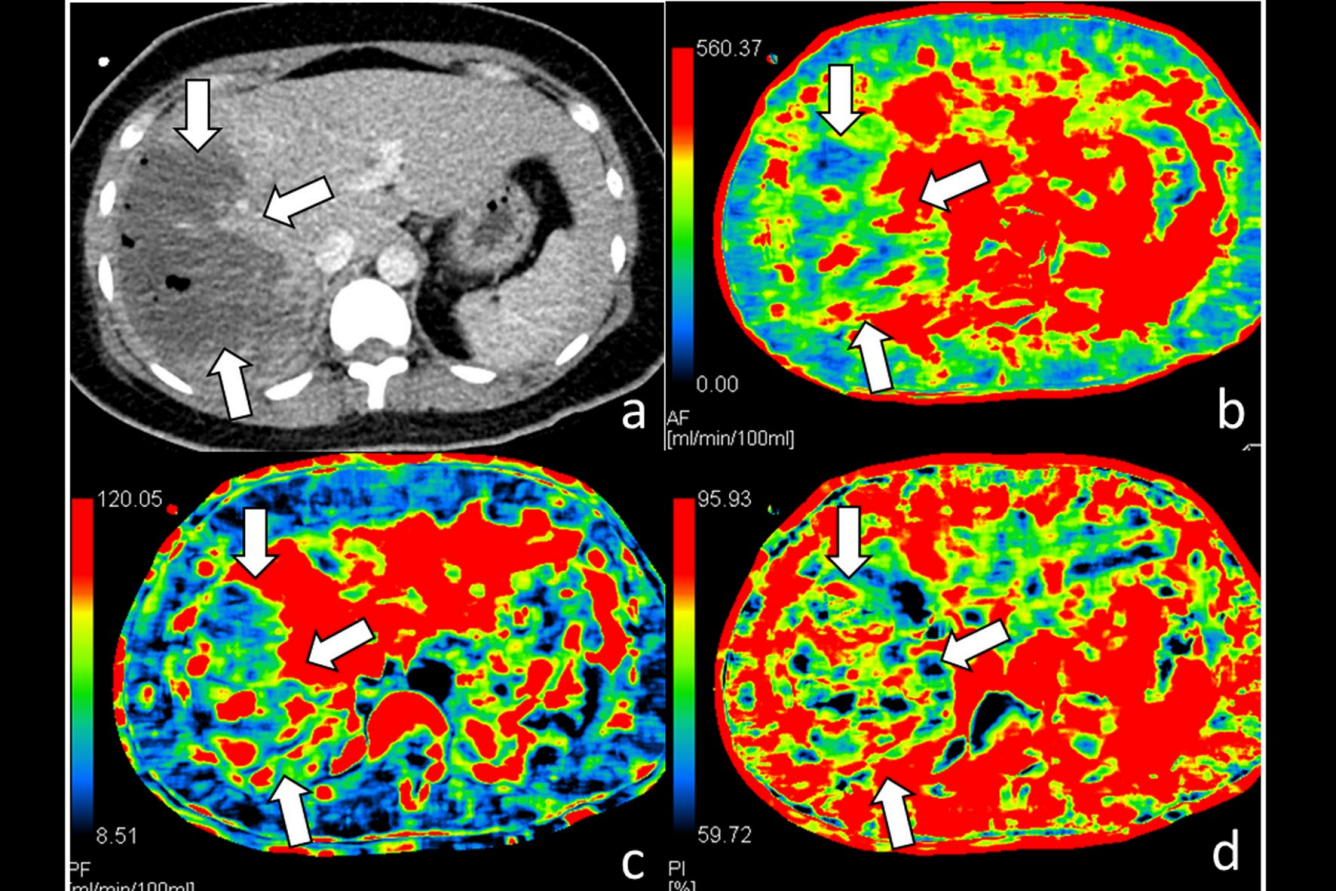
CT of a 34-year-old woman with grade IV liver injury at right lobe after embolization management. (a) Contrast-enhanced CT of the liver shows extensive right lobe injury (arrows); (b) hepatic arterial perfusion CT and (c) hepatic portal venous perfusion CT show decreased arterial and portal venous flows to traumatic parenchyma at right lobe (arrows); (d) hepatic perfusion index which is a ratio of arterial perfusion to the total hepatic perfusion is normal at traumatic parenchyma (arrows).
